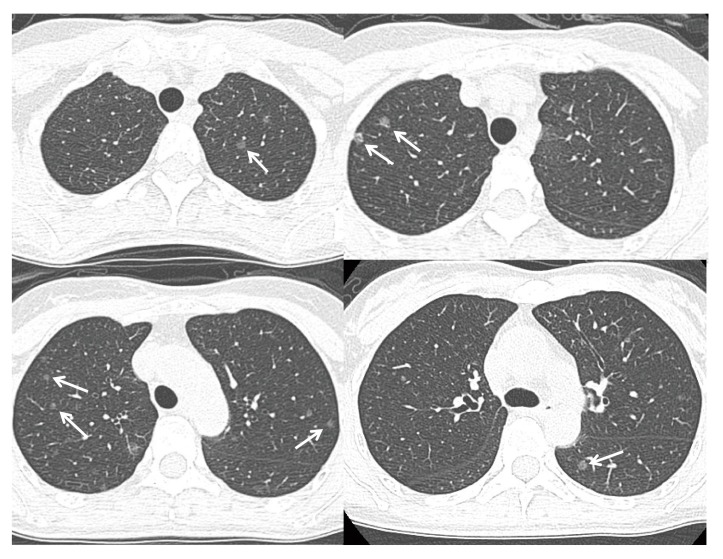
A 48 year-old non-smoking woman was found to have multiple subcentimetre ground glass opacities (arrows) in her bilateral lungs on a low-dose CT screening. Video-assisted thoracoscopic surgery with a right upper lobe wedge resection confirmed the diagnosis of synchronous multiple lung adenocarcinomas harboring the EGFR wild-type.
